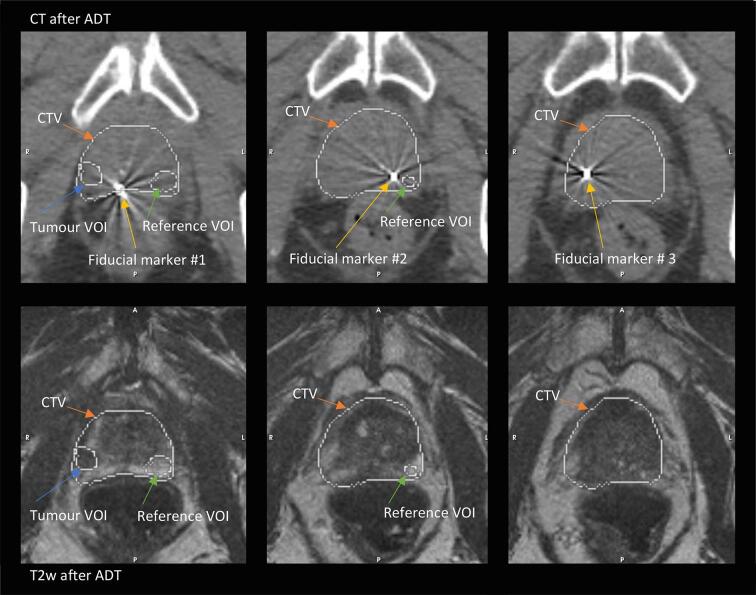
Fiducial markers in CT (top row) and T2w MRI (bottom row) after ADT in three different locations. Fiducial markers are inserted after ADT. VOIs indicate the tumour VOI and reference VOI registered with r + DIR techniques from ADC at baseline.
